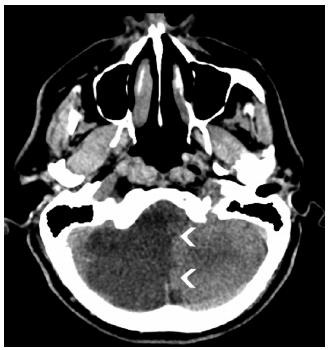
Patient 5. Axial unenhanced computed tomography - hypoattenuating area involving the posteroinferior right cerebellum (arrowheads), related to ischemic stroke involving posteroinferior cerebellar artery.
